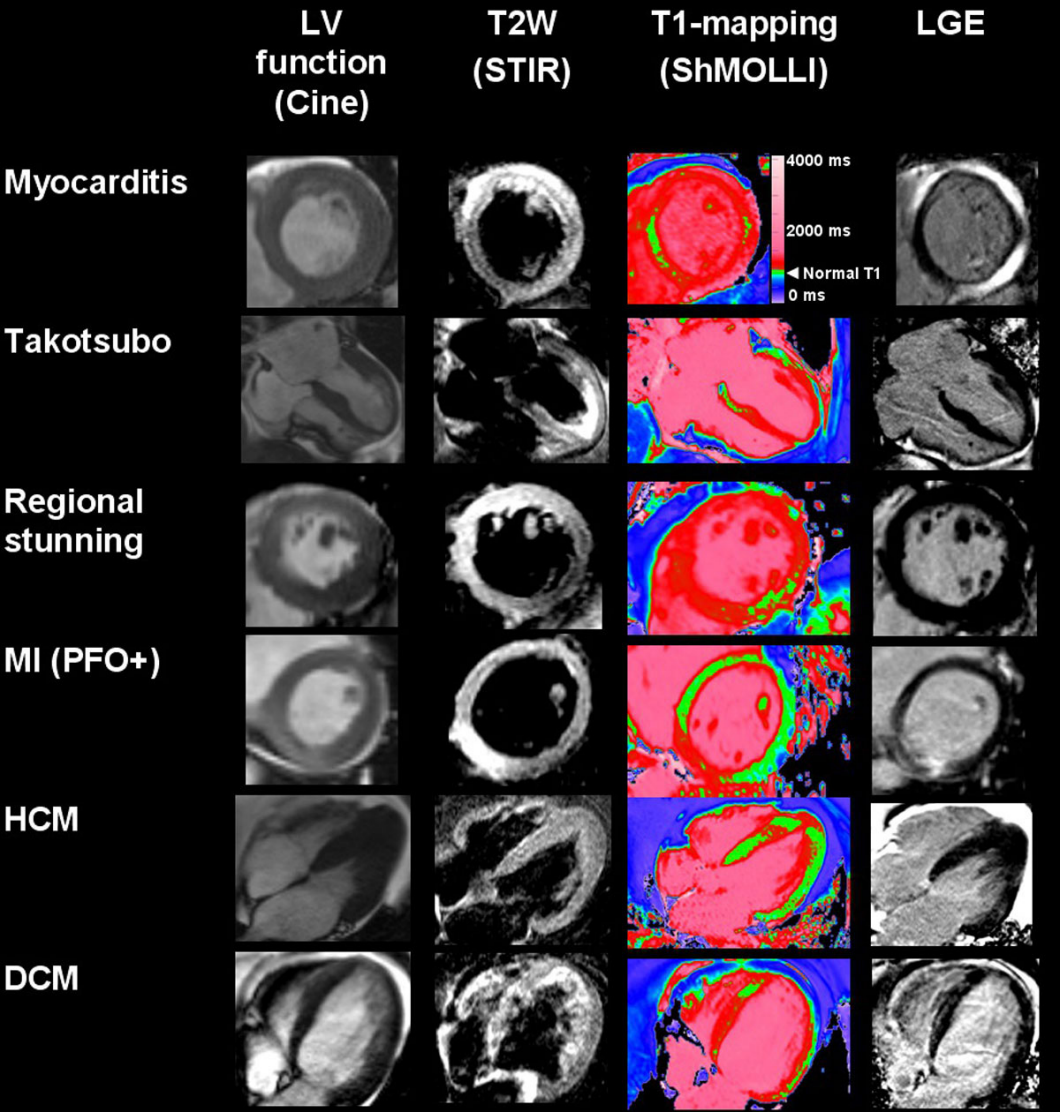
Early CMR using multiparametric tissue characterization including cine, T2-weighted (T2W), T1-mapping and late gadolinium enhancement imaging to assess patients presenting with acute chest pain, positive troponins and non-obstructive coronary arteries. On T1-maps, green areas within the LV denote normal myocardium; red areas have a T1> 990 ms, consistent with acute injury.
